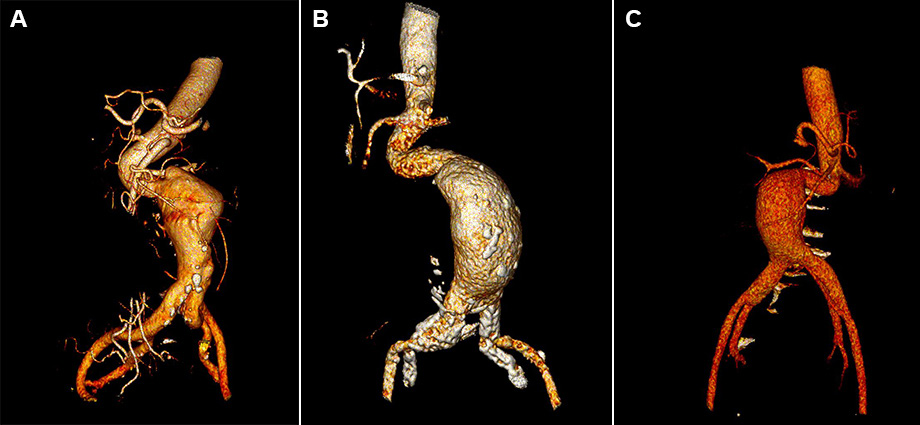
Preoperative 3D CT angiography reconstructions showing tortuous aortic neck. (A) 3D CT reconstruction of the first patient; (B) 3D CT reconstruction of the second patient; (C) 3D CT reconstruction of the third patient.
